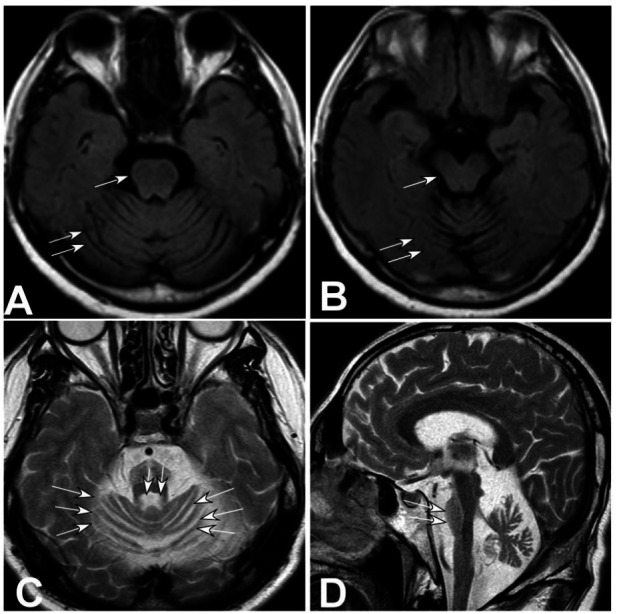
Magnetic resonance imaging (MRI) of the brain in case 2 showing atrophy of the cerebellum, brainstem, pons, and projective fibers. (A and B). Top view: cerebellar atrophy (double arrows) and thinning of the brainstem (arrow). (C). Top view: decreased signal (atrophy) at the anterior edge of the pons (double arrows) and the projection fibers (arrows). (D). Lateral view demonstrated atrophy of the pons (double arrows).
